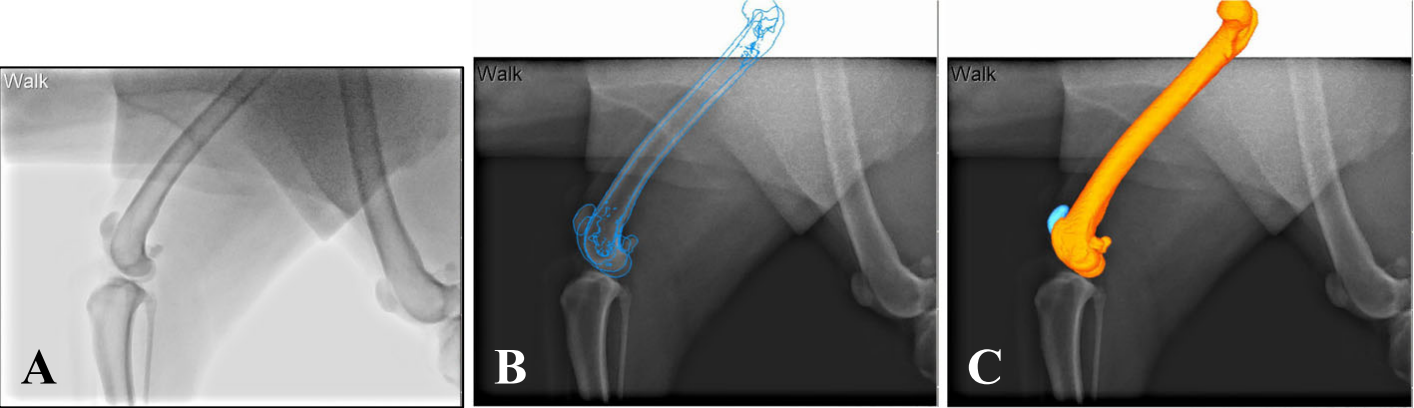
Shape-matching process. a Flat-panel radiographic image imported into JointTrack. b Contoured silhouettes based on the 3-D models of the femur and patella utilized for shape-matching over the radiographic image. c Shape-matched 3-D projections of the femur and patella
